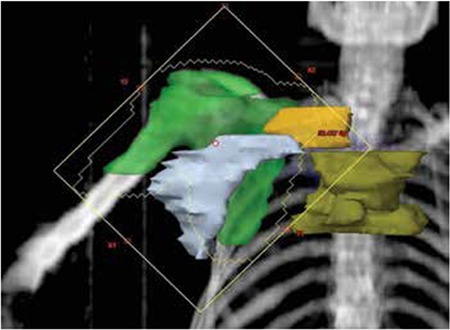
Digitally reconstructed radiograph of the scapula field including axillary lymph nodes, supraclavicular lymph nodes, and the upper mediastinal lymph node area.
